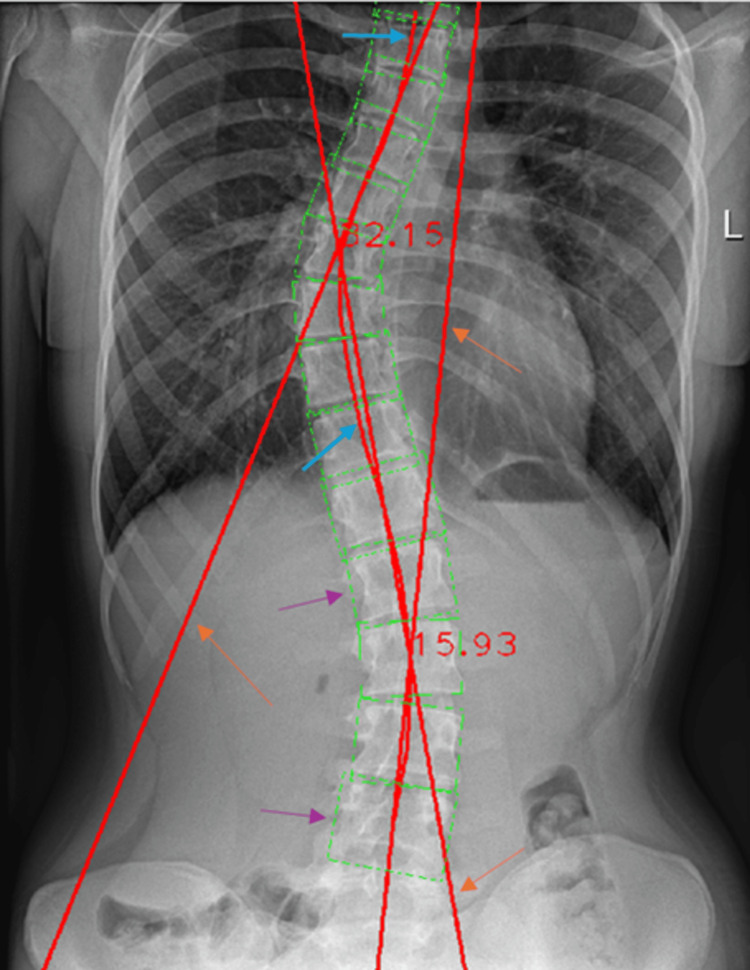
Resulting elaborated X-ray image. 1) Orange arrows showing the tangent lines from which the Cobb angle is calculated, 2) purple arrows showing the vertebral boxes, 3) light-blue arrows showing the central spinal line calculated via the polynomial curve fitting.
